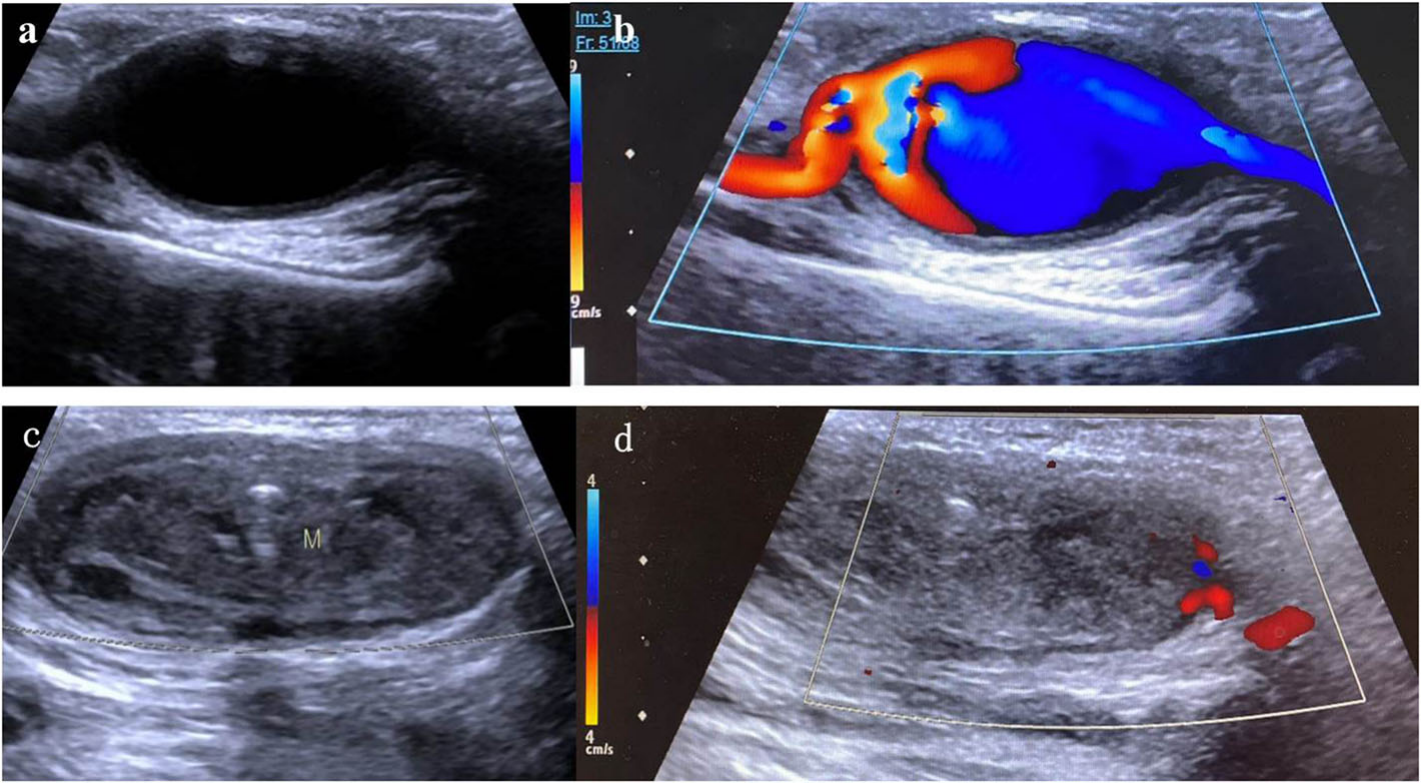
The ultrasound images of bilateral axillary artery aneurysm. a, b The right giant aneurysm (a without color Doppler; b with color Doppler) without thrombus, with the flow velocity of input and output site of the aneurysm 0.8 m/s and 0.6 m/s respectively; c, d The left giant aneurysm with massive thrombus occlusion (c without color Doppler; d with color Doppler) and sparse blood flow near the mass
